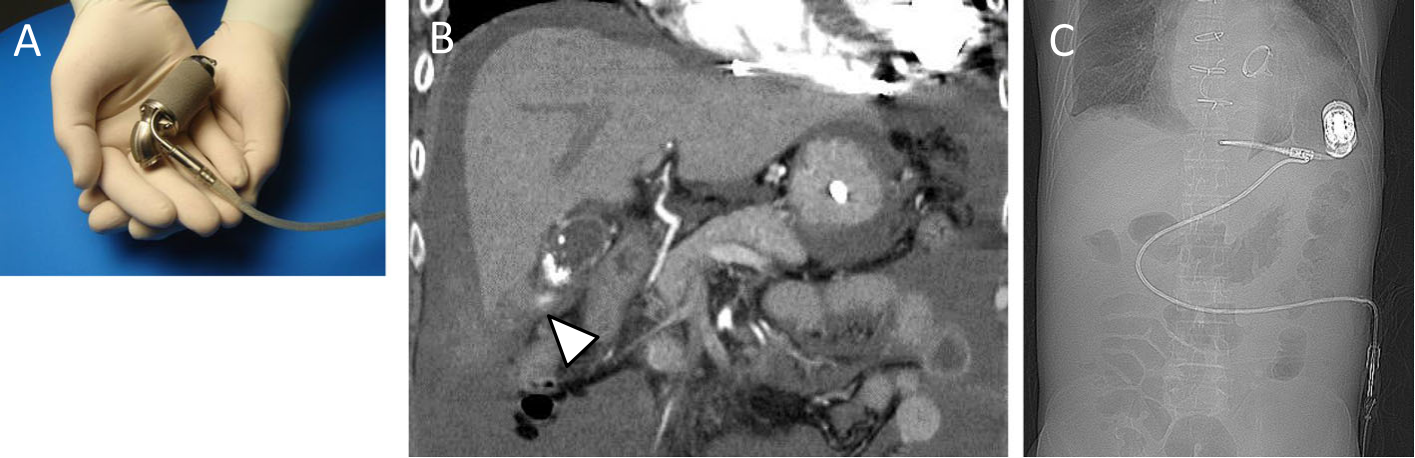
Diagnostic images and a photograph of LVAD. a JarvikTM 2000. b Abdominal radiographs taken on admission showing the LVAD main body and driveline location. c Coronal computed tomography scan showing a high-density area near the gallbladder (arrowhead), high-density ascites, and gallbladder stone. There is no high-density area in the gallbladder
